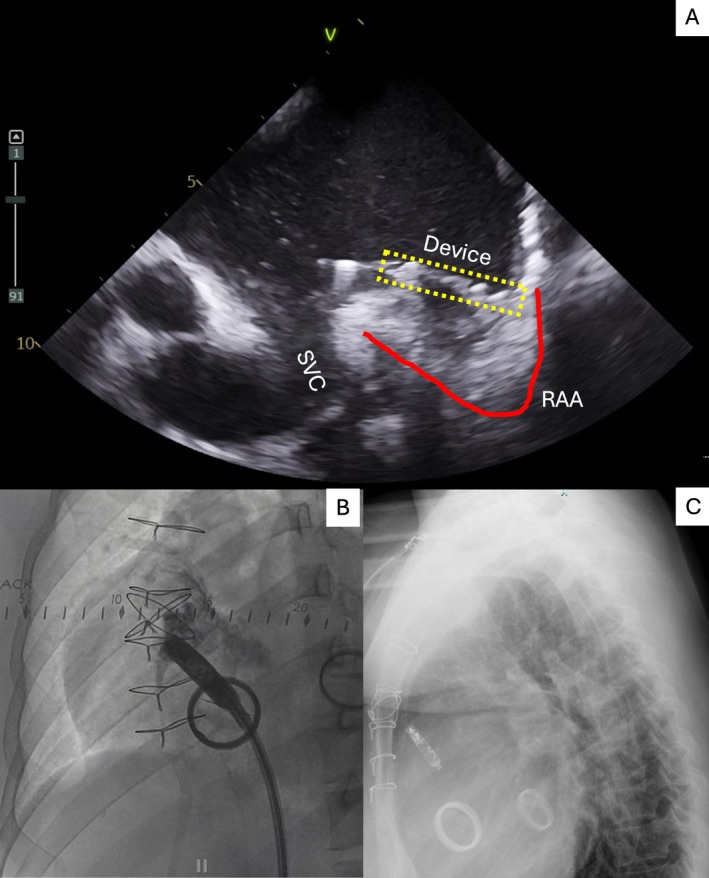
Intracardiac echocardiography (A), fluoroscopy (B), and chest radiography (C) confirming device positioning.
